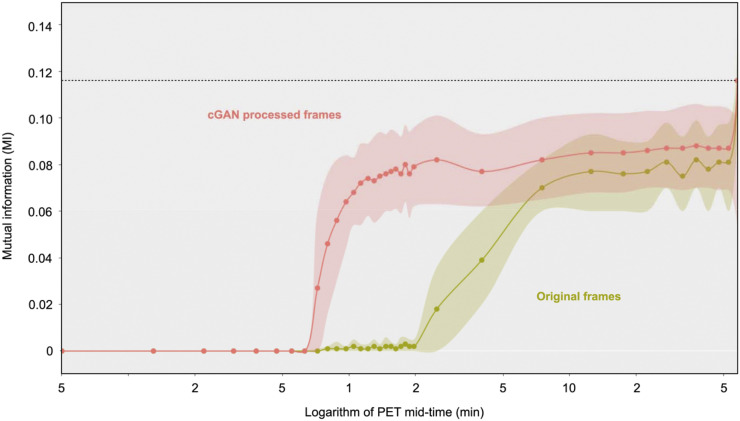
Comparison of MI index between the original (green) and cGAN processed (red) dynamic frames with the reference frame as a (log) function of scan time. Note that neither dynamic sequence is motion corrected. Values represent the average and the SD (shown as shaded area) of the test dataset (*n* = 6). cGAN processing increases MI of ^18^F-FDG brain images with respect to the reference frame close to the MI of late frames (dotted line).
